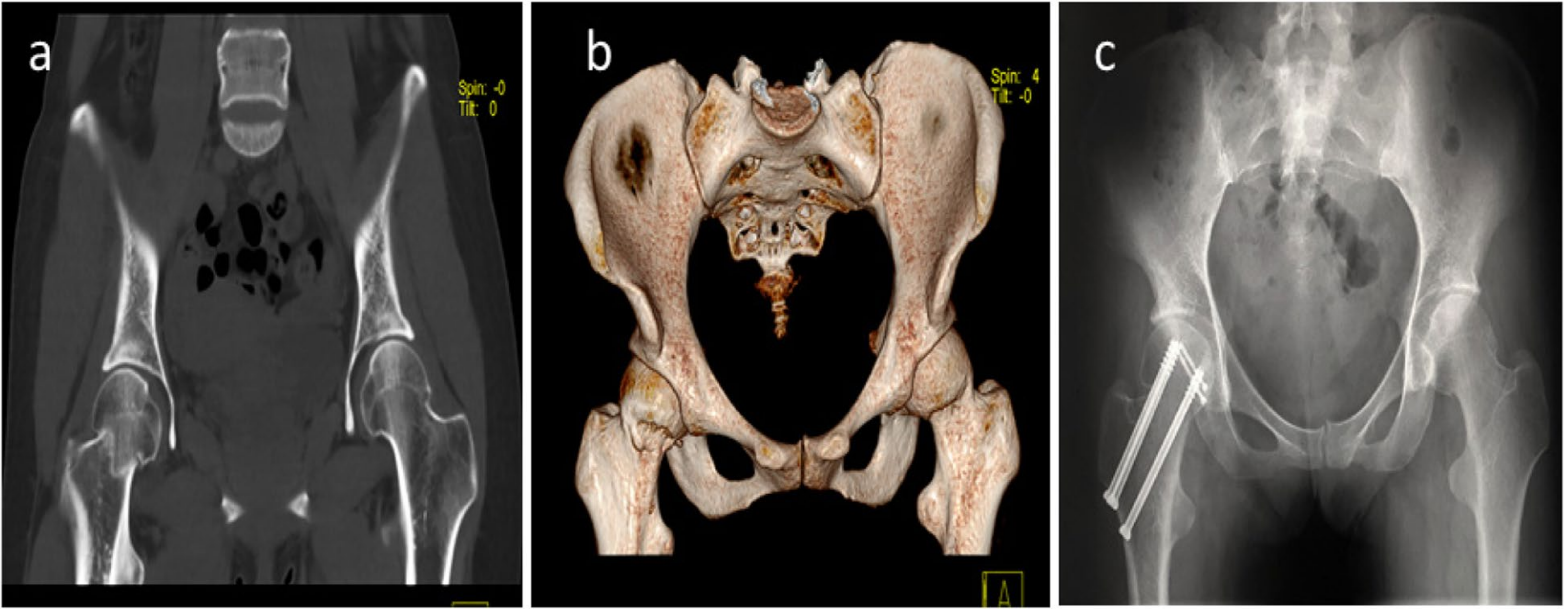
A 27-year female sustained injury after motor vehicle accident. a Coronal view b 3D CT demonstrated right sided Pipkin III femoral head and neck fracture. c The fracture was anatomically reduced with herbert screw via anterior approach and femoral neck was corrected with three cannulated screw utilizing lateral stab approach
